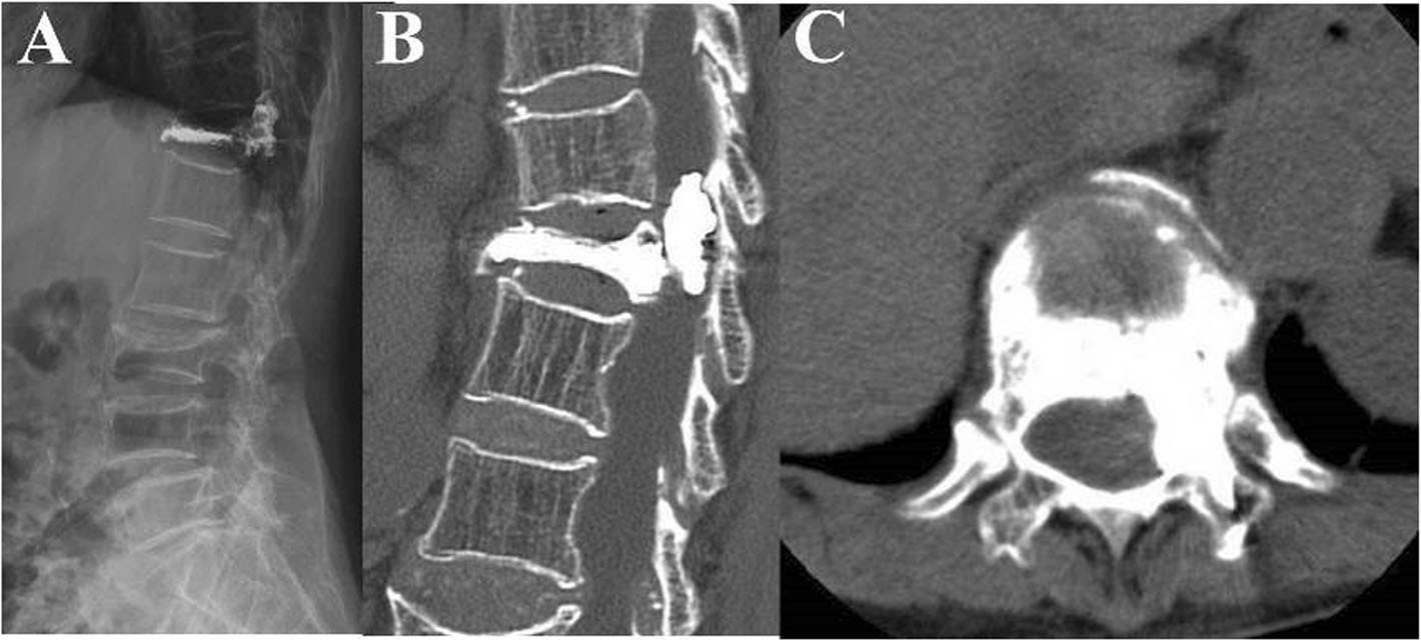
An 89-year-old female developed severe back pain and left-side lower limb motor weakness after T12 PVP using PMMA cement. Postoperative lateral (a) and CT (b) showing a type-C posterior leakage and cement leakage into the left spinal canal, which caused left-side lower limb weakness (c). The neurological deficit was fully recovered after immediate decompression surgery
